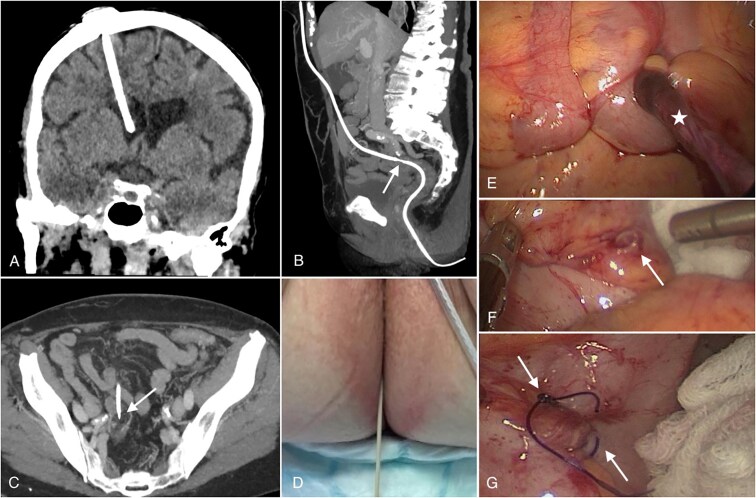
(A): Coronal reconstruction of cranial computed tomography (CT) shows the intraventricular location of the ventriculoperitoneal shunt tip in the frontal horn of the right lateral ventricle. (B): Sagittal maximum intensity projection (MIP) reconstruction of an abdominal CT scan showing the subcutaneous and later intraperitoneal positioning of the shunt before perforating the sigmoid colon (white arrow). (C) Shows the perforation site on an axial abdominal CT scan (marked with a white arrow). (D) Reveals the protrusion of the catheter. (E, F, and G): Laparoscopic pictures showing the catheter (white star in E) as well as the perforation site (diverticulum) in the sigmoid before (arrow in F) and after removal and surgical repair of the perforation site (arrow in G).
